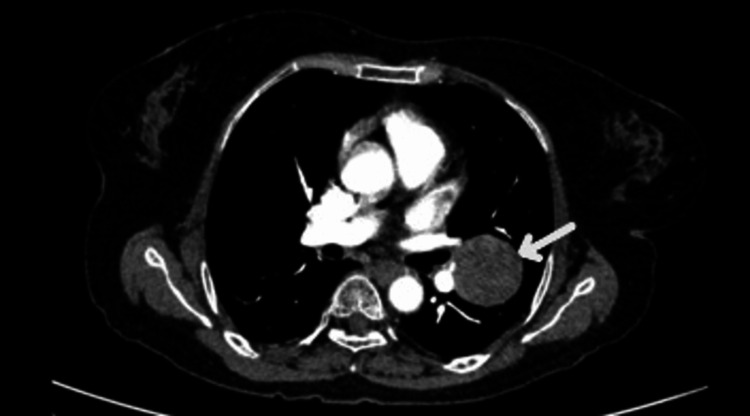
Contrast-enhanced computed tomography of the thorax axial section (mediastinal window) showing a well-defined smoothly marginated heterogeneously enhancing soft tissue density lesion in the left upper lobe
